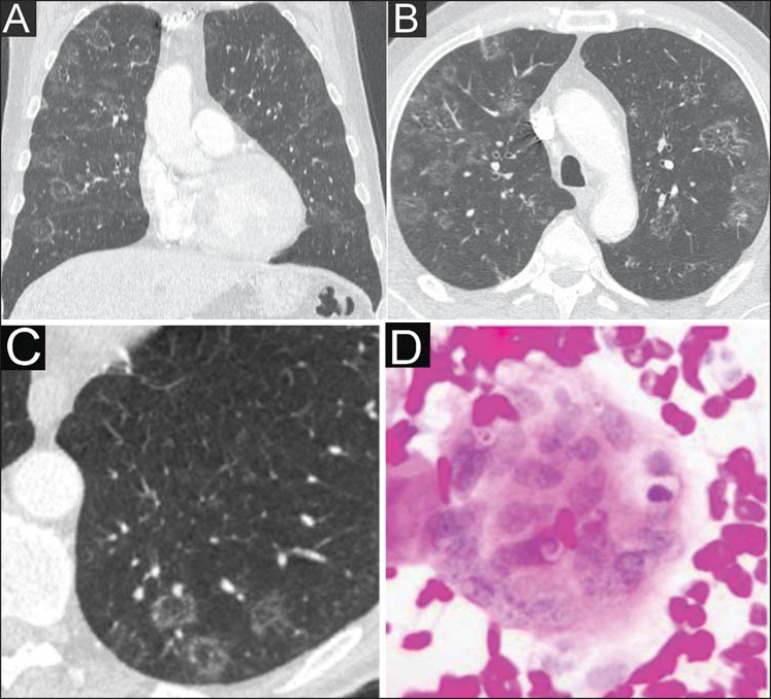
A,B,C: Computed tomography sections showing, principally, focal
